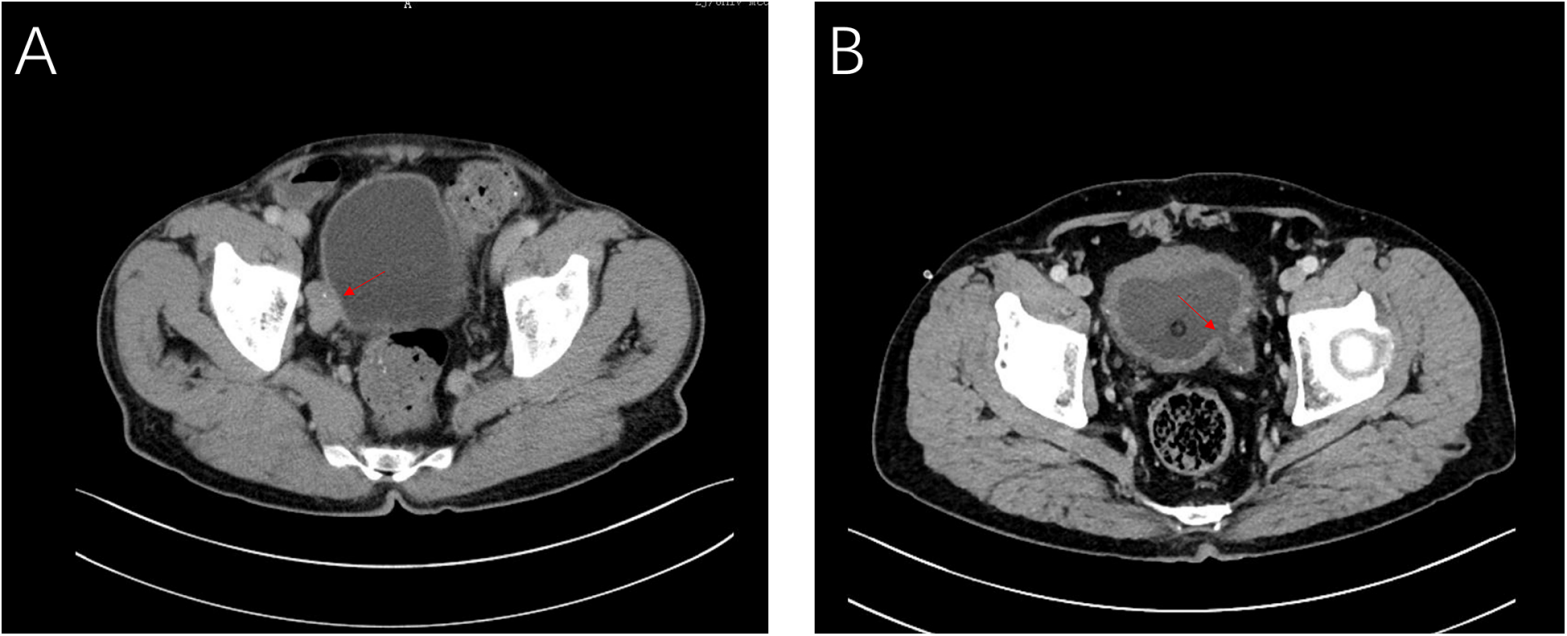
CT images of case 1 (A) and case 2 (B) showing the intradiverticular bladder tumor noted with arrow.
